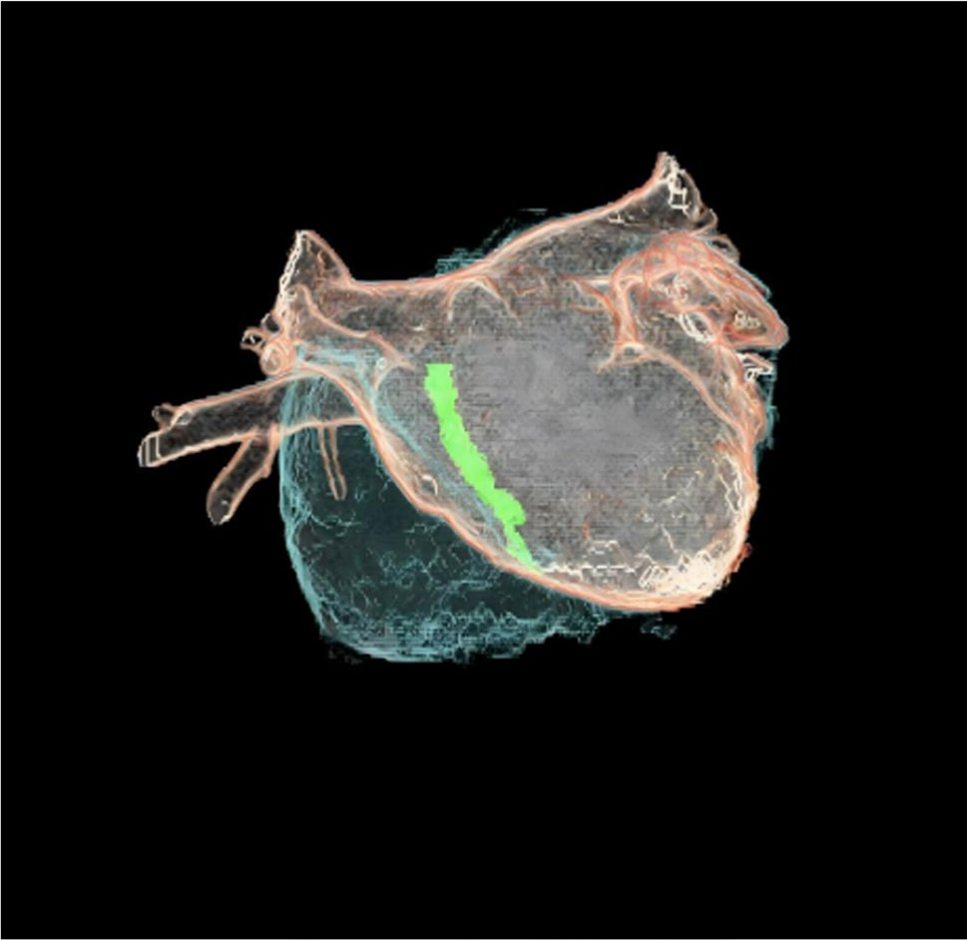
CT atrial reconstruction revealed the anatomical relationship between the PFO and the atria
